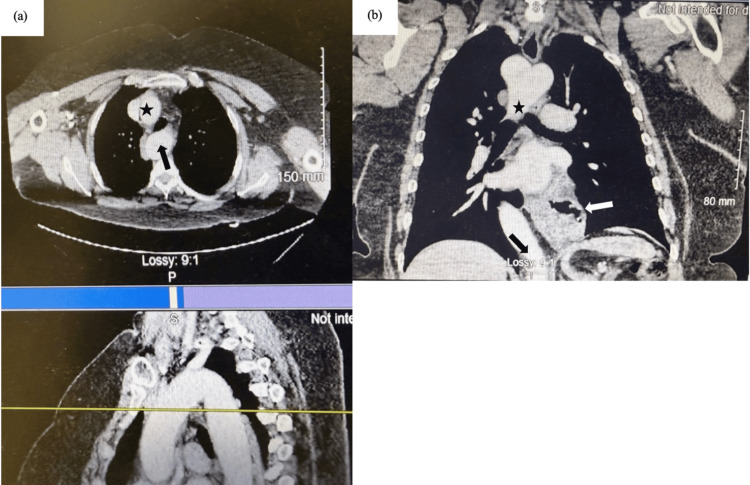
CT chest of patient with RAA and PEH (a) Ascending aorta (star) and RAA (arrow) traversing the right main bronchus. (b) Right-sided descending aorta (star) before crossing midline at the level of the diaphragm (black arrow). PEH extending into the thoracic cavity (white arrow). CT: computed tomography, RAA: right aortic arch, PEH: paraesophageal hiatal hernia
